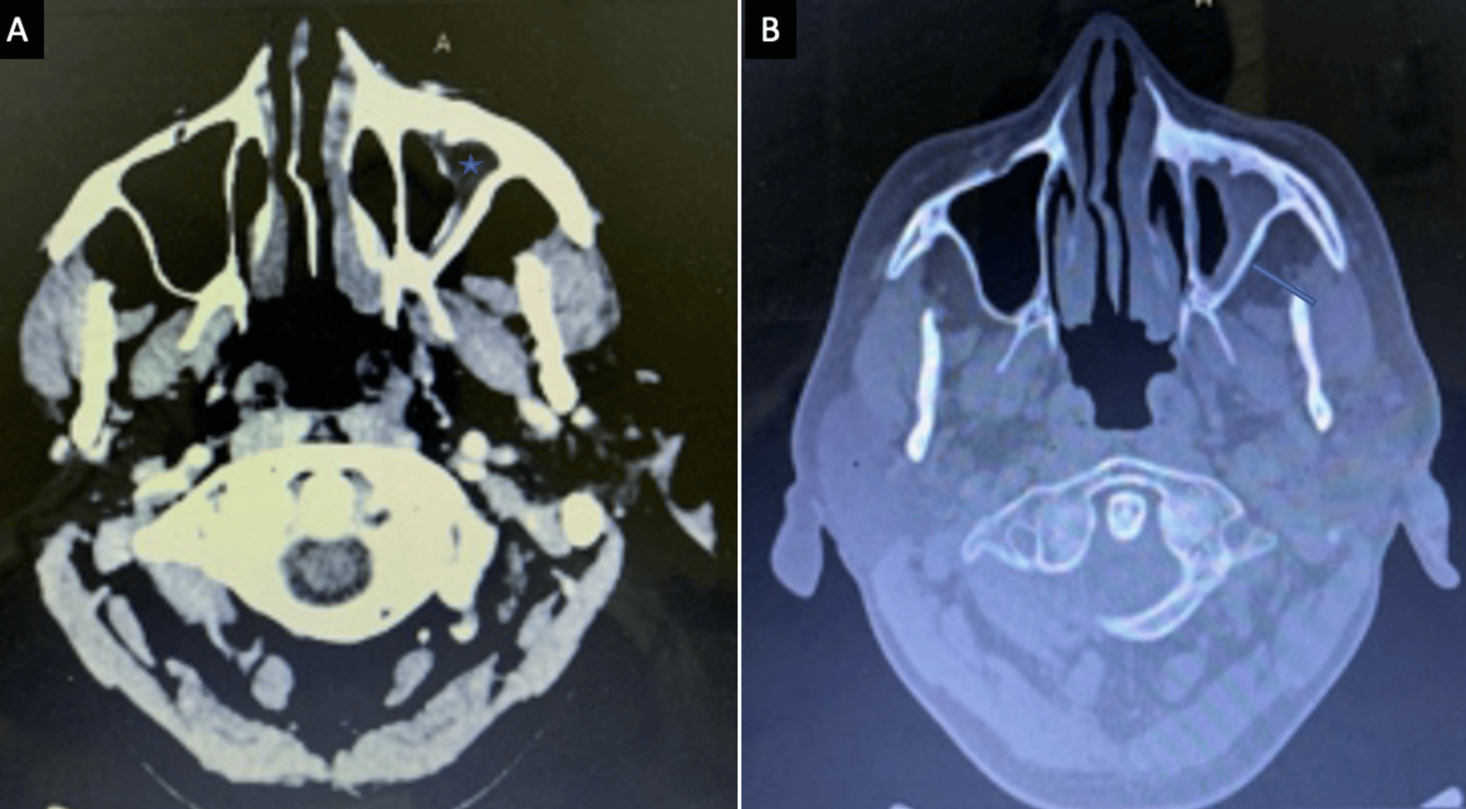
(A) A case of chronic invasive fungal infection after six months of medical treatment showing residual mucosal thickening in the left maxillary sinus (*) (CT scan, axial). (B) CT scan bone window showing sclerosis of sinus walls (↗) after six months of medical treatment (CT scan, bone window). CT: computed tomography
